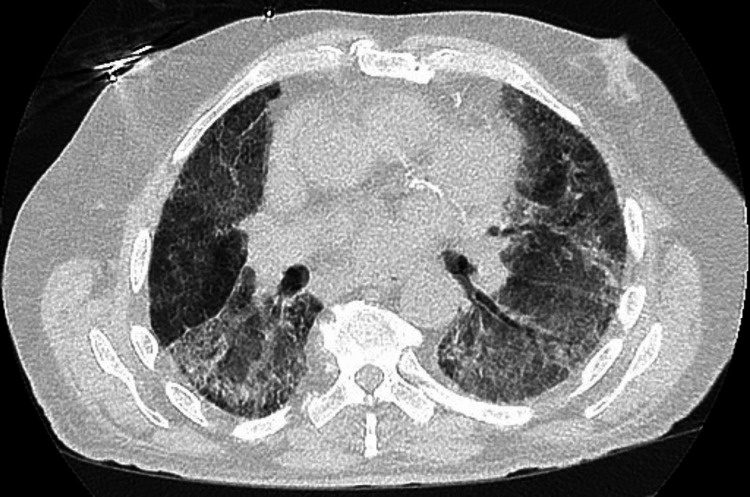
CT scan on admission showing multifocal multilobar ground-glass opacities.
